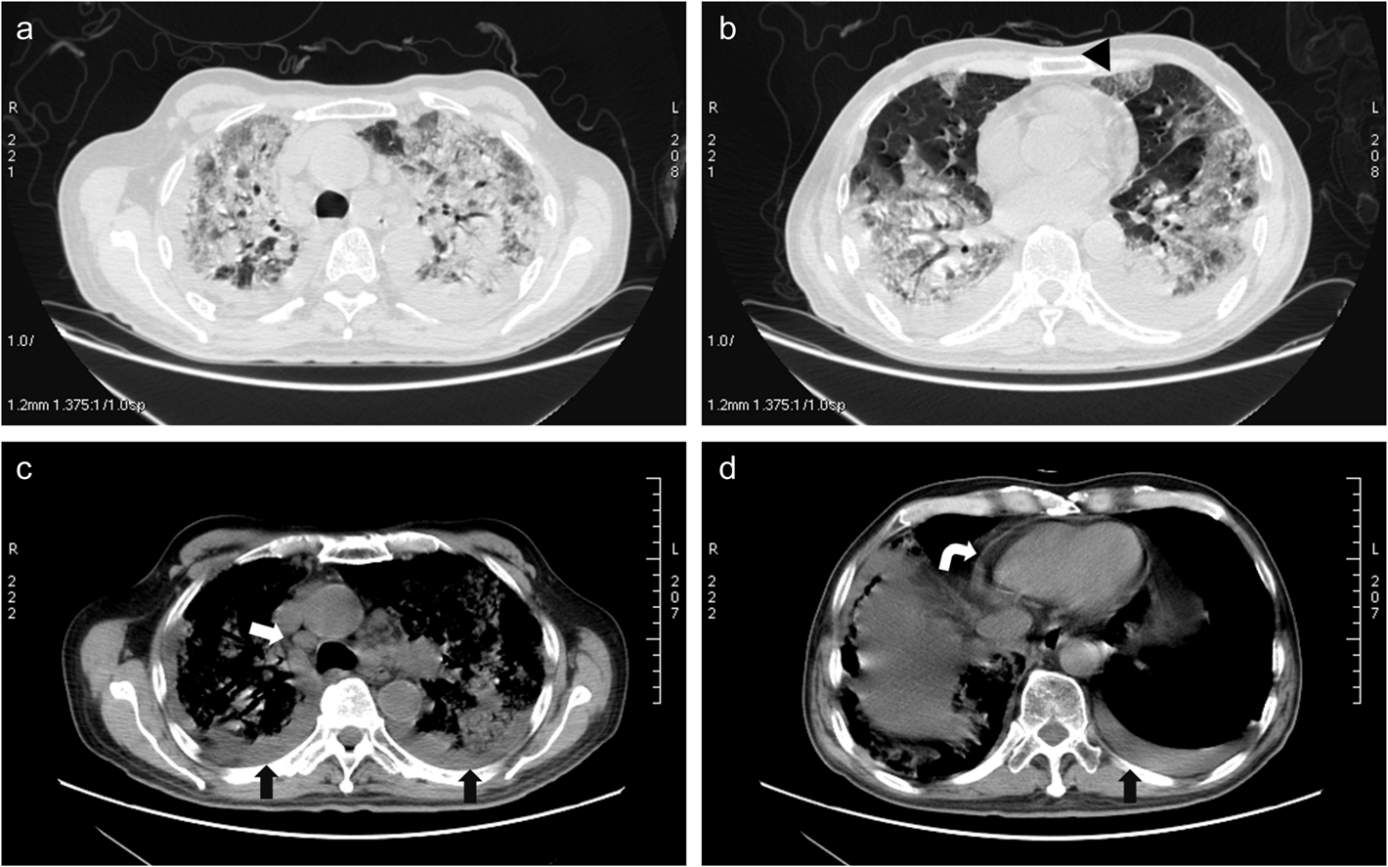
Non-contrast enhanced thin-section CT scans on day 4 after symptom onset. a, b Lung window, bilateral ground-glass opacities with clear boundary, multifocal consolidations, air bronchograms, smooth interlobular and intralobular septal thickening could be seen (black triangle). c, d Mediastinum window, lymphadenopathy (white arrow), bilateral pleural effusions (black arrows), and moderate pericardial effusions (curved white arrow) were shown
